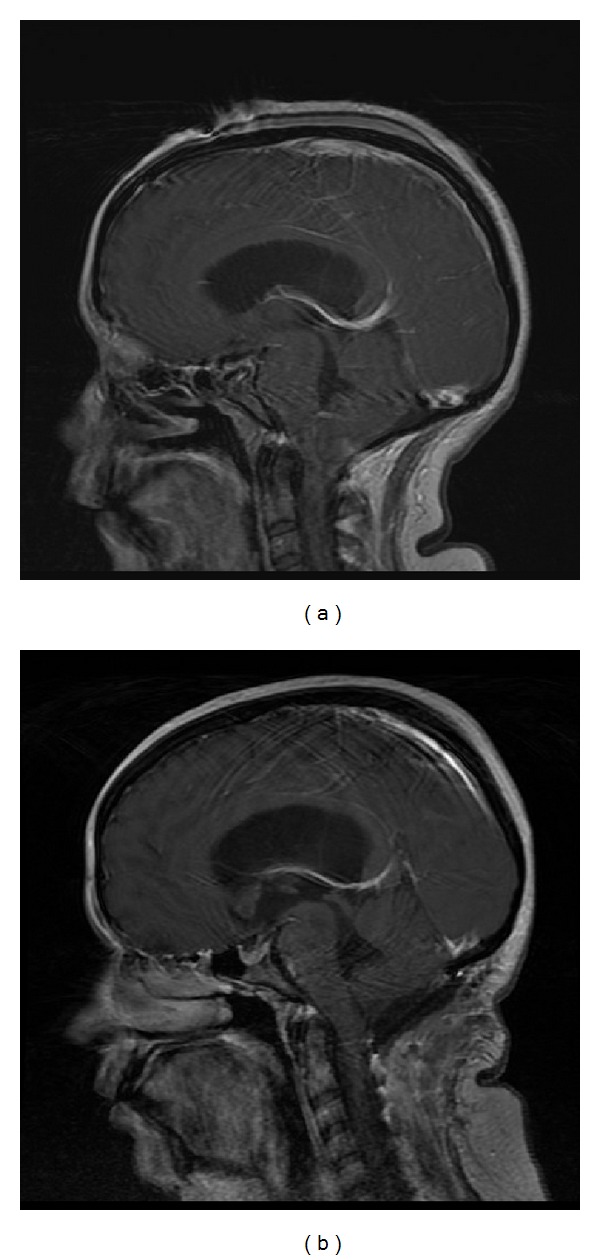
(a) Preoperative T_1_-weighted MRI with sagittal view. (b) Postoperative T_1_-weighted MRI with sagittal view.
